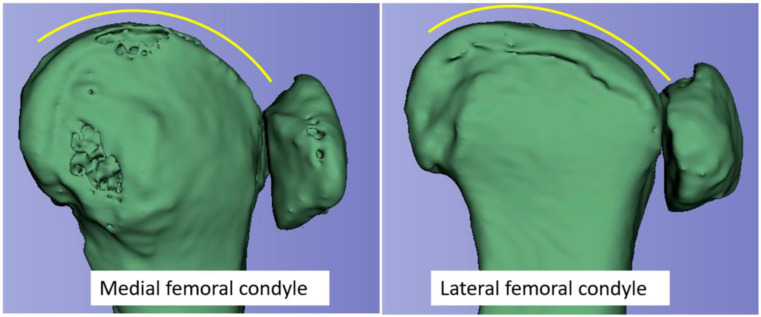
3D animation of the medial and lateral femoral condyles of a knee joint from a CT scan. Note the different shapes of the condyles in the anterior and the transition area to the distal region.
